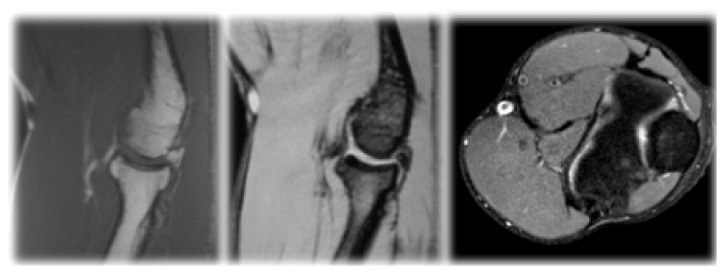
MRI of patients right elbow at one-year follow-up, demonstrating full recovery of the previously described lesions.
